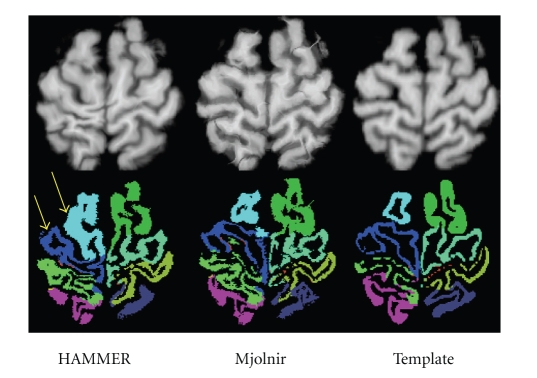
Example of an outlier occurring in HAMMER, where HAMMER's ability to follow the folding pattern of the cortex is lacking. The top row shows the deformed MR images and the corresponding template image on the right. The bottom row shows the corresponding deformed labels from the NIREP Na0 database and the template's labels.
